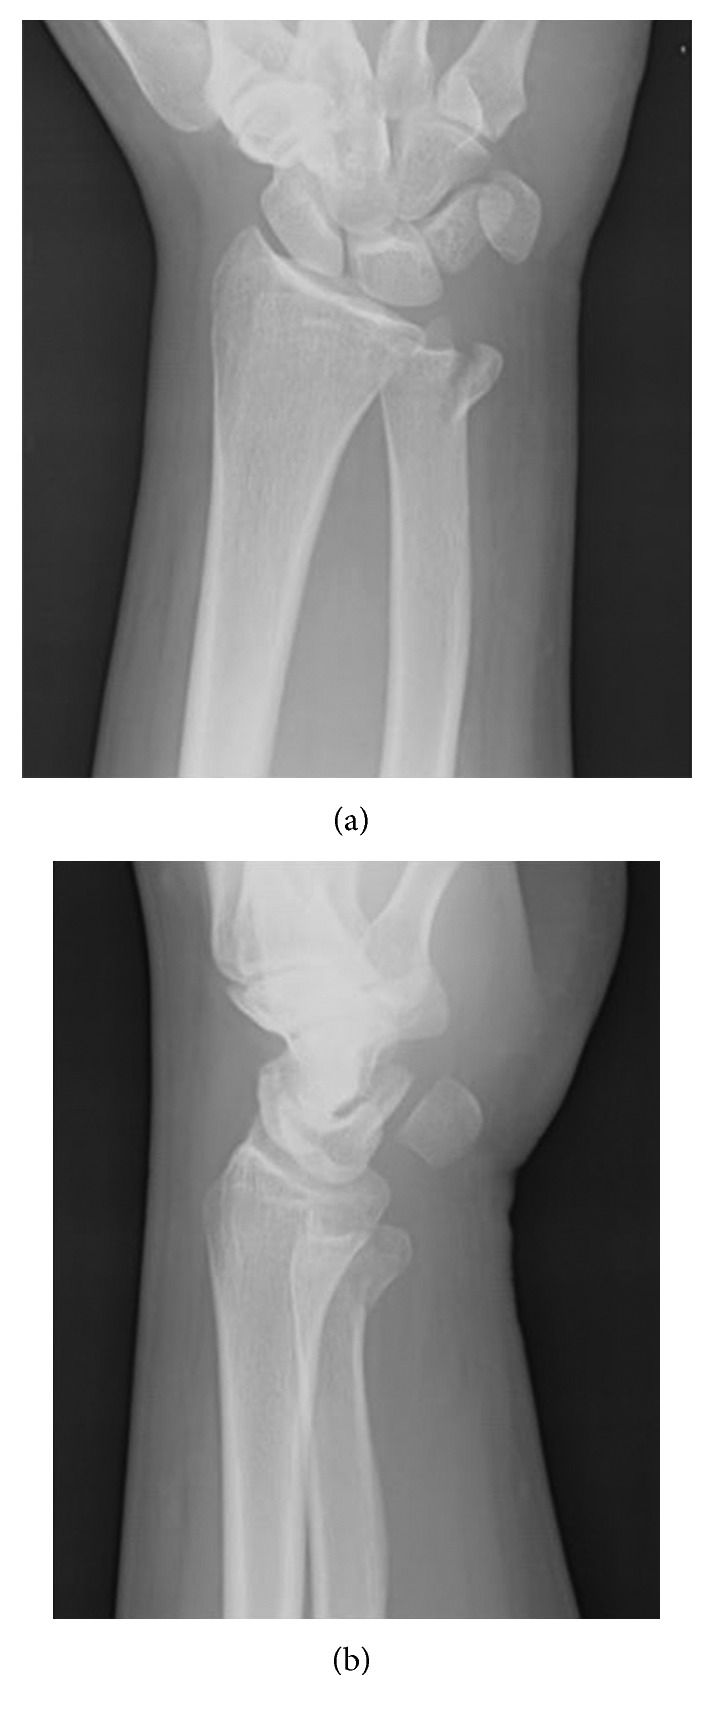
Preoperative radiographs. (a) Anterior-posterior view and (b) lateral view.
